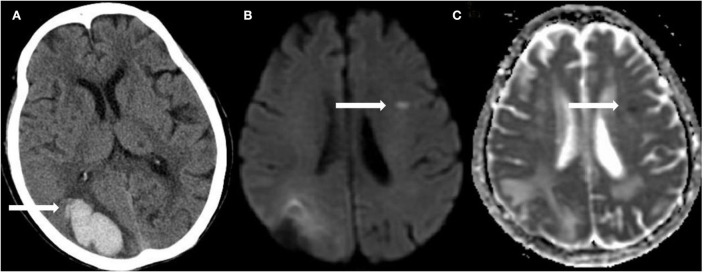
A representative example of R-DWILs. In a 92-year-old female with right occipital lobe hemorrhage (A), diffusion-weighted imaging (DWI) shows a small remote ischemic lesion on the left frontal lobe (B), with corresponding low signal intensity in the apparent diffusion coefficient (ADC) map (C).
